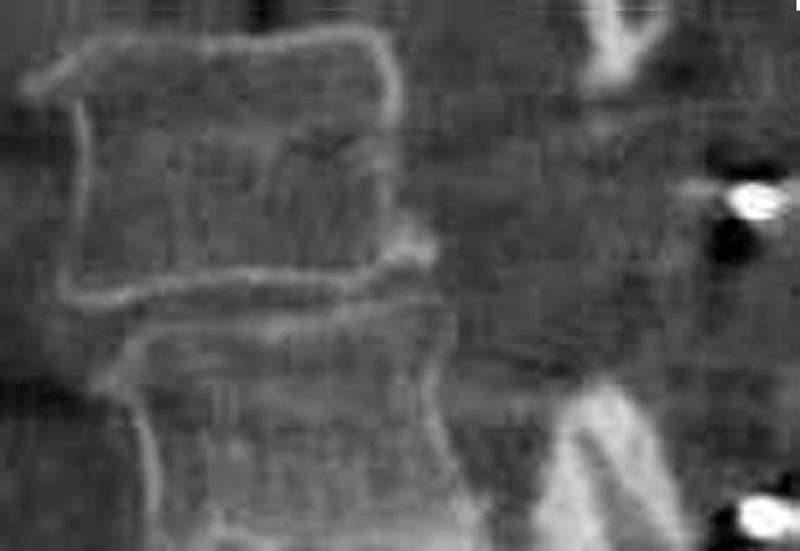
Pre-operative CT scan showing L2 to L3 degeneration and stenosis adjacent to previous L3 to S1 fusion.
